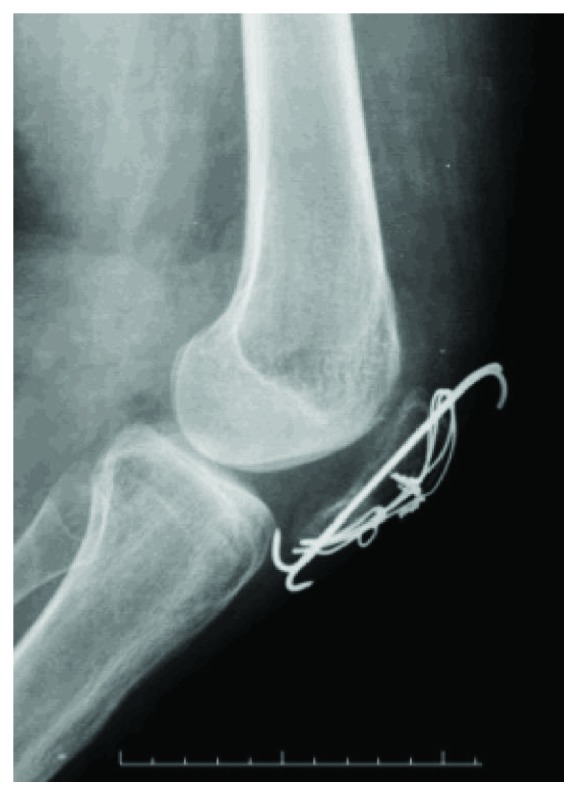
Radiograph obtained during postoperative month 3.
